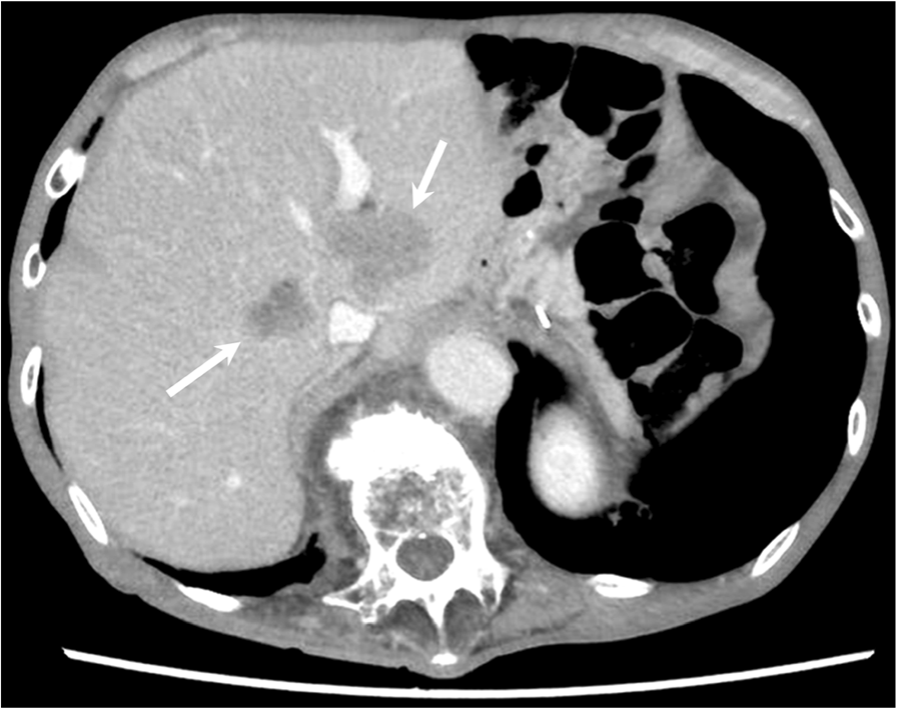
Initial findings on abdominal computed tomography (CT). Abdominal enhanced-contrast CT revealed multiple liver metastases in the bilateral lobe of the liver (arrows)
